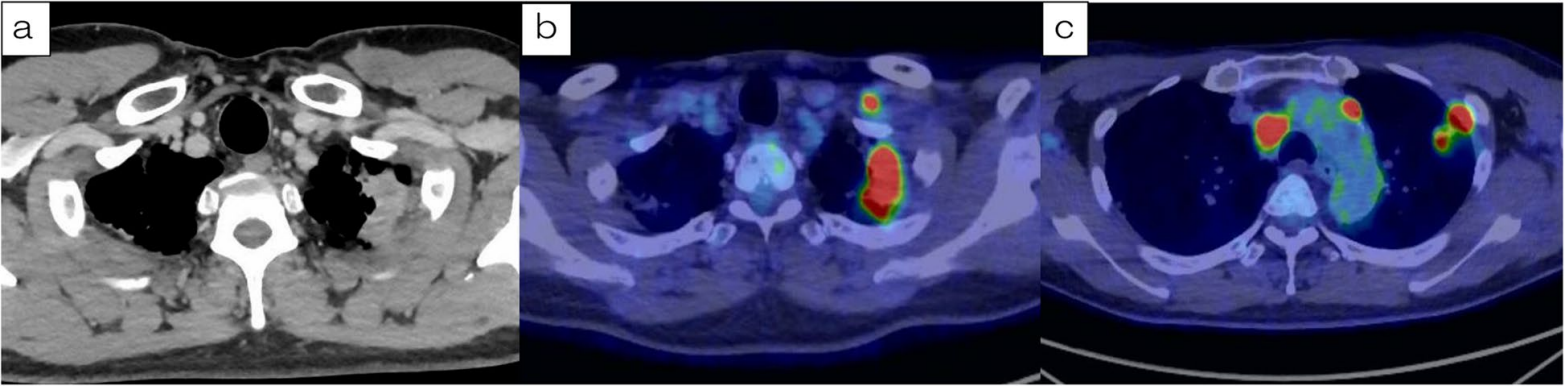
Computed tomography (CT) and positron emission tomography (PET) before chemoradiotherapy (CRT) followed by durvalumab. a CT at the initial diagnosis revealed a tumor of 3.1 cm in diameter originating from the left upper lobe in the left superior sulcus that was in contact with the chest wall and enlarged hilar, periaortic, and left supraclavicular lymph nodes. b, c Fluorodeoxyglucose (FDG)-PET revealed uptake in the primary lesion, left supraclavicular lymph nodes, mediastinal lymph nodes, and periaortic lymph nodes. The maximum standardized uptake value on FDG-PET in the primary lesion was 14.1
